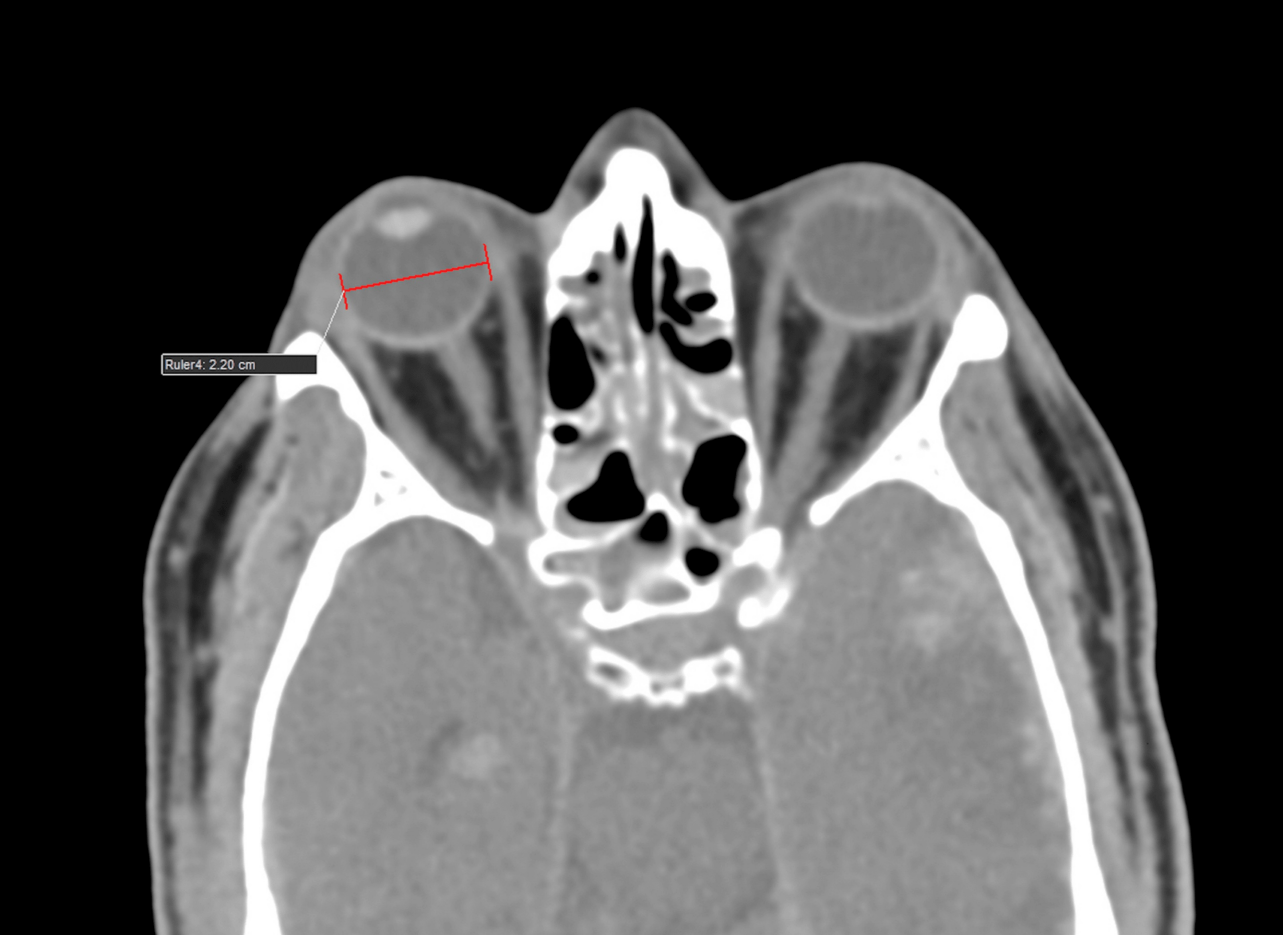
A 55-year-old male who was involved in a road traffic accident and sustained a head injury presented with a Glasgow Coma Scale (GCS) of 7. The initial computed tomography (CT) scan of the brain (axial section, 1 mm slice thickness, standard mediastinum algorithm with a window level of 10 Hounsfield units {HU} and a width of 300 HU) shows an eyeball transverse diameter (ETD) of 22 mm (from sclera to sclera), indicating severe traumatic brain injury (TBI).
